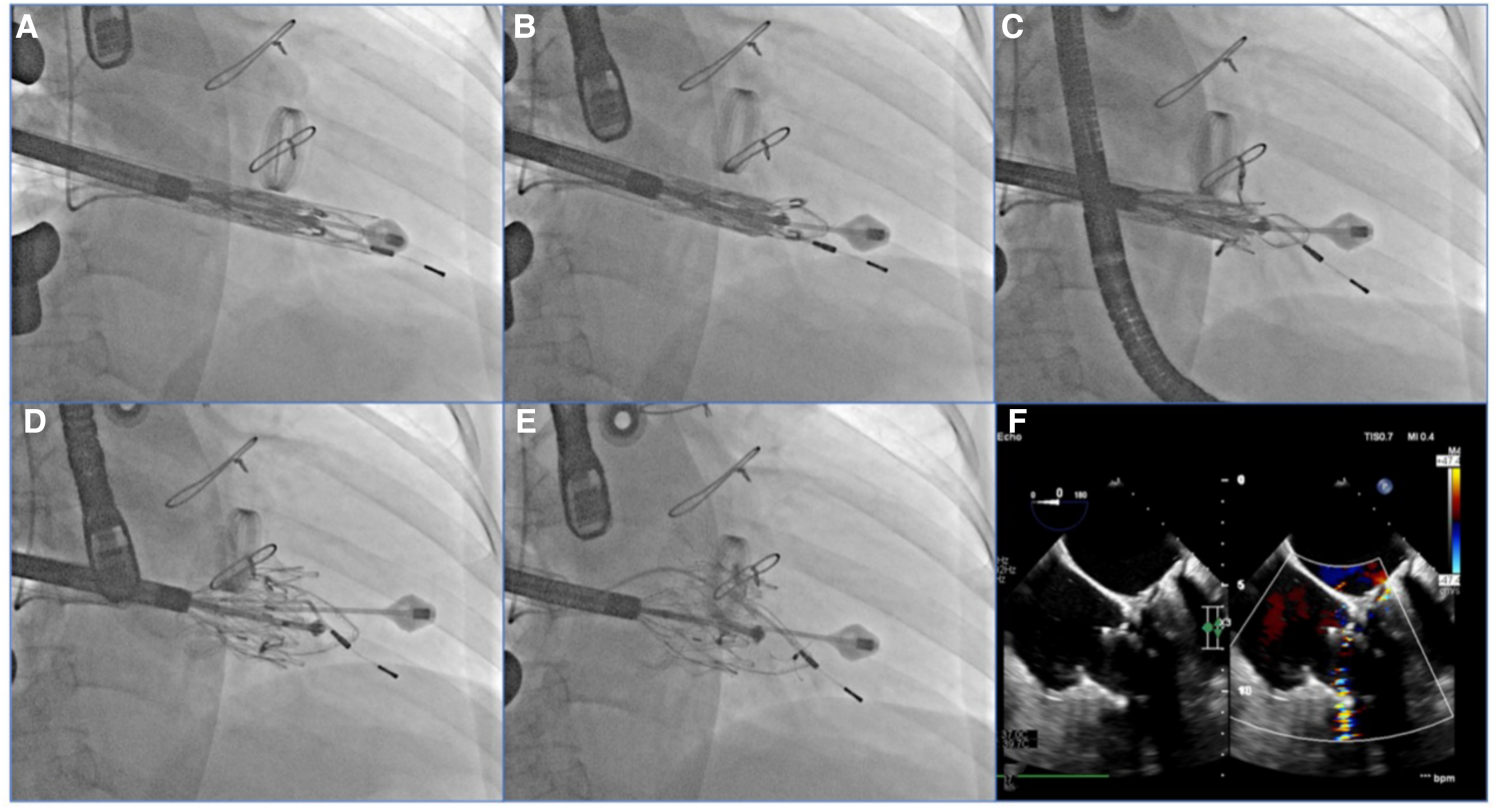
TEE and DSA were guided for TTVR. (A) DSA showed severe TR on TV. (B) The LuX-Valve system guided by TEE was used to deliver the prosthesis to the tricuspid annulus through the right intercostal approach. (C) The delivery system first released IVA and two graspers, and TEE guided the anterior leaflet of the tricuspid valve to be clamped by the grasper. (D) The annulus skirt and atrium disc were released in turn, and the position of the implant was adjusted by TEE guidance to ensure that there was no obvious paravalvular leakage. (E) The prosthesis was completely released after fixation with IVA. (F) Postoperative TEE showed that TR disappeared immediately. TEE, transesophageal echocardiography; DSA, digital subtracted angiography; TR, tricuspid regurgitation; TV, tricuspid valve; IVA, interventricular anchor.
